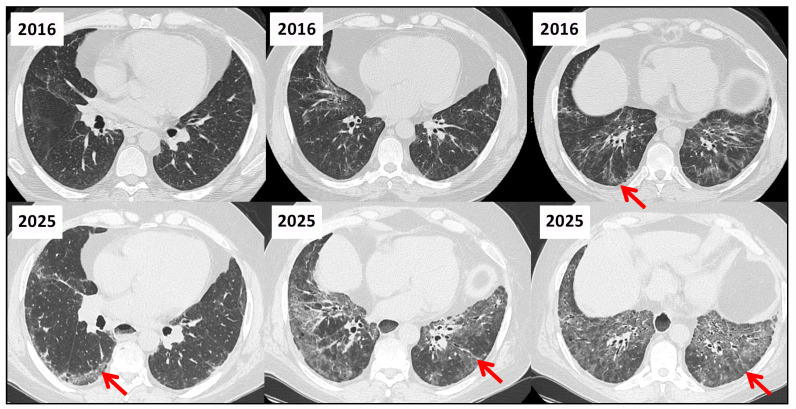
Serial HRCT Scans in a 53-Year-Old Patient with Systemic Sclerosis and ILD with a NSIP Pattern (2016–2025). Axial HRCT images acquired in 2016 (top row) and 2025 (bottom row) demonstrate radiological progression of fibrotic NSIP in a patient with systemic sclerosis. In 2016, the scans reveal bilateral, symmetric reticular opacities with peripheral and basal predominance, mild traction bronchiectasis, and relative subpleural sparing—features consistent with fibrotic NSIP. Nine years later, the 2025 images show a marked increase in reticulation and traction bronchiectasis (red arrows). There is visible lower lobe volume loss and architectural distortion, indicating progression of fibrotic remodeling. These findings reflect a worsening fibrotic phenotype over time, emphasizing the chronic and progressive nature of SSc-associated ILD. Mild to moderate dilatation of the esophagus is visible, more prominently in the 2025 images, consistent with progressive esophageal involvement typical of systemic sclerosis. The presence of intraluminal gas and increased luminal diameter suggests dysmotility, which may contribute to chronic aspiration and exacerbate pulmonary disease progression in SSc.
